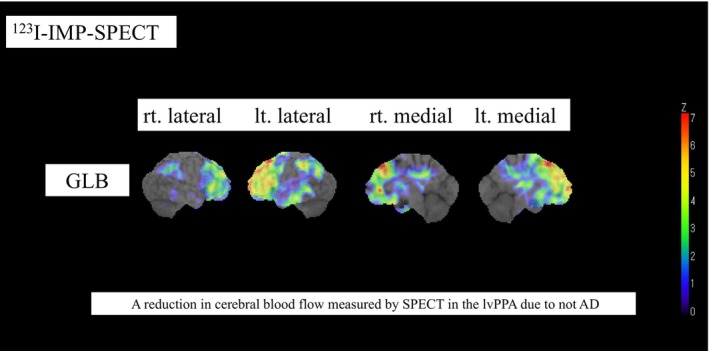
Reduction in cerebral blood flow (rCBF) measured by SPECT in the patients with lvPPA not due to AD. A reduction in cerebral blood flow (rCBF) measured by SPECT in patients with lvPPA not due to AD is shown using 3D‐SSP. The SPECT results displayed rCBF in the frontal lobes, which is an atypical pattern for AD.
